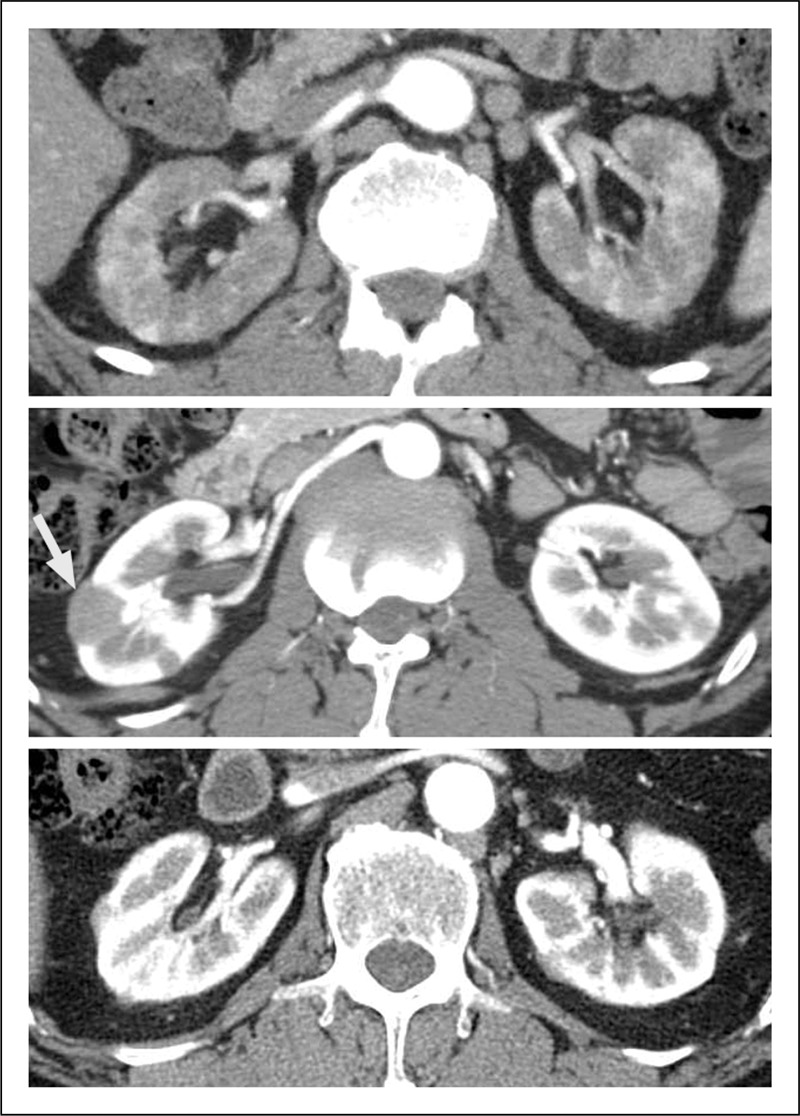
A variety of patterns of multiple low-density lesions on contrast-enhanced computed tomography (CT). Upper: Contrast-enhanced CT scan shows bilateral diffuse patchy involvement. Middle: Contrast-enhanced CT scan shows multiple parenchymal low-density lesions including mass-like lesions protruding beyond the surface of the kidney (arrow). Lower: Contrast-enhanced CT scan shows a rim-like lesion of the kidney.
